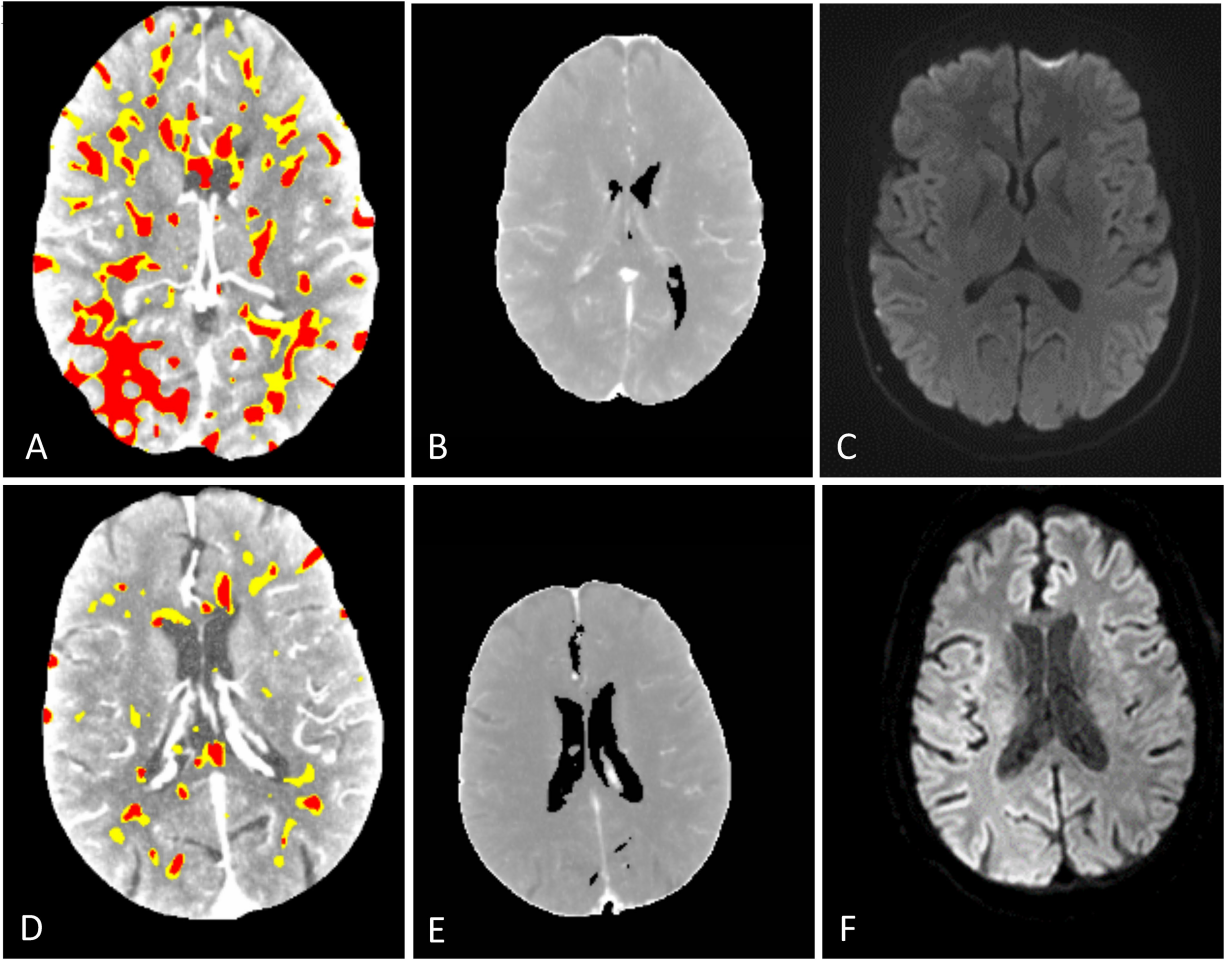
Representative CTP output maps of syngo.via (A,D) showing diffuse hypoperfused (yellow) and infarct core (red) areas. CMN hyperperfusion/core maps (B,E) show no infarct core. Corresponding MRI DWI (C,F) images show no signs of acute infarction
